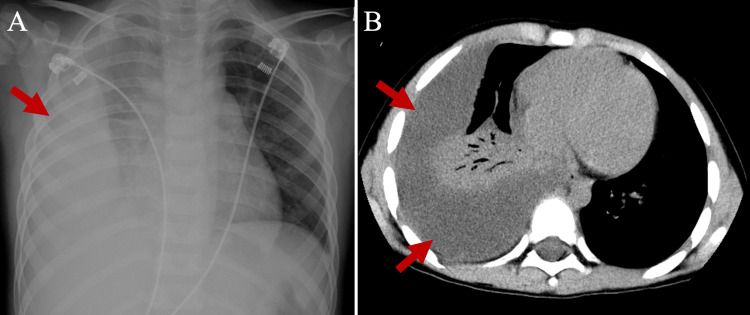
Pre-treatment chest radiography and computed tomography examinations Pre-treatment chest radiograph demonstrated decreased radiopacity in the right lung field, indicative of potential pleural effusion (A). Pre-treatment chest computed tomography revealed significant accumulation of pleural effusion fluid in the right pleural cavity and atelectasis of the right lung (B).
